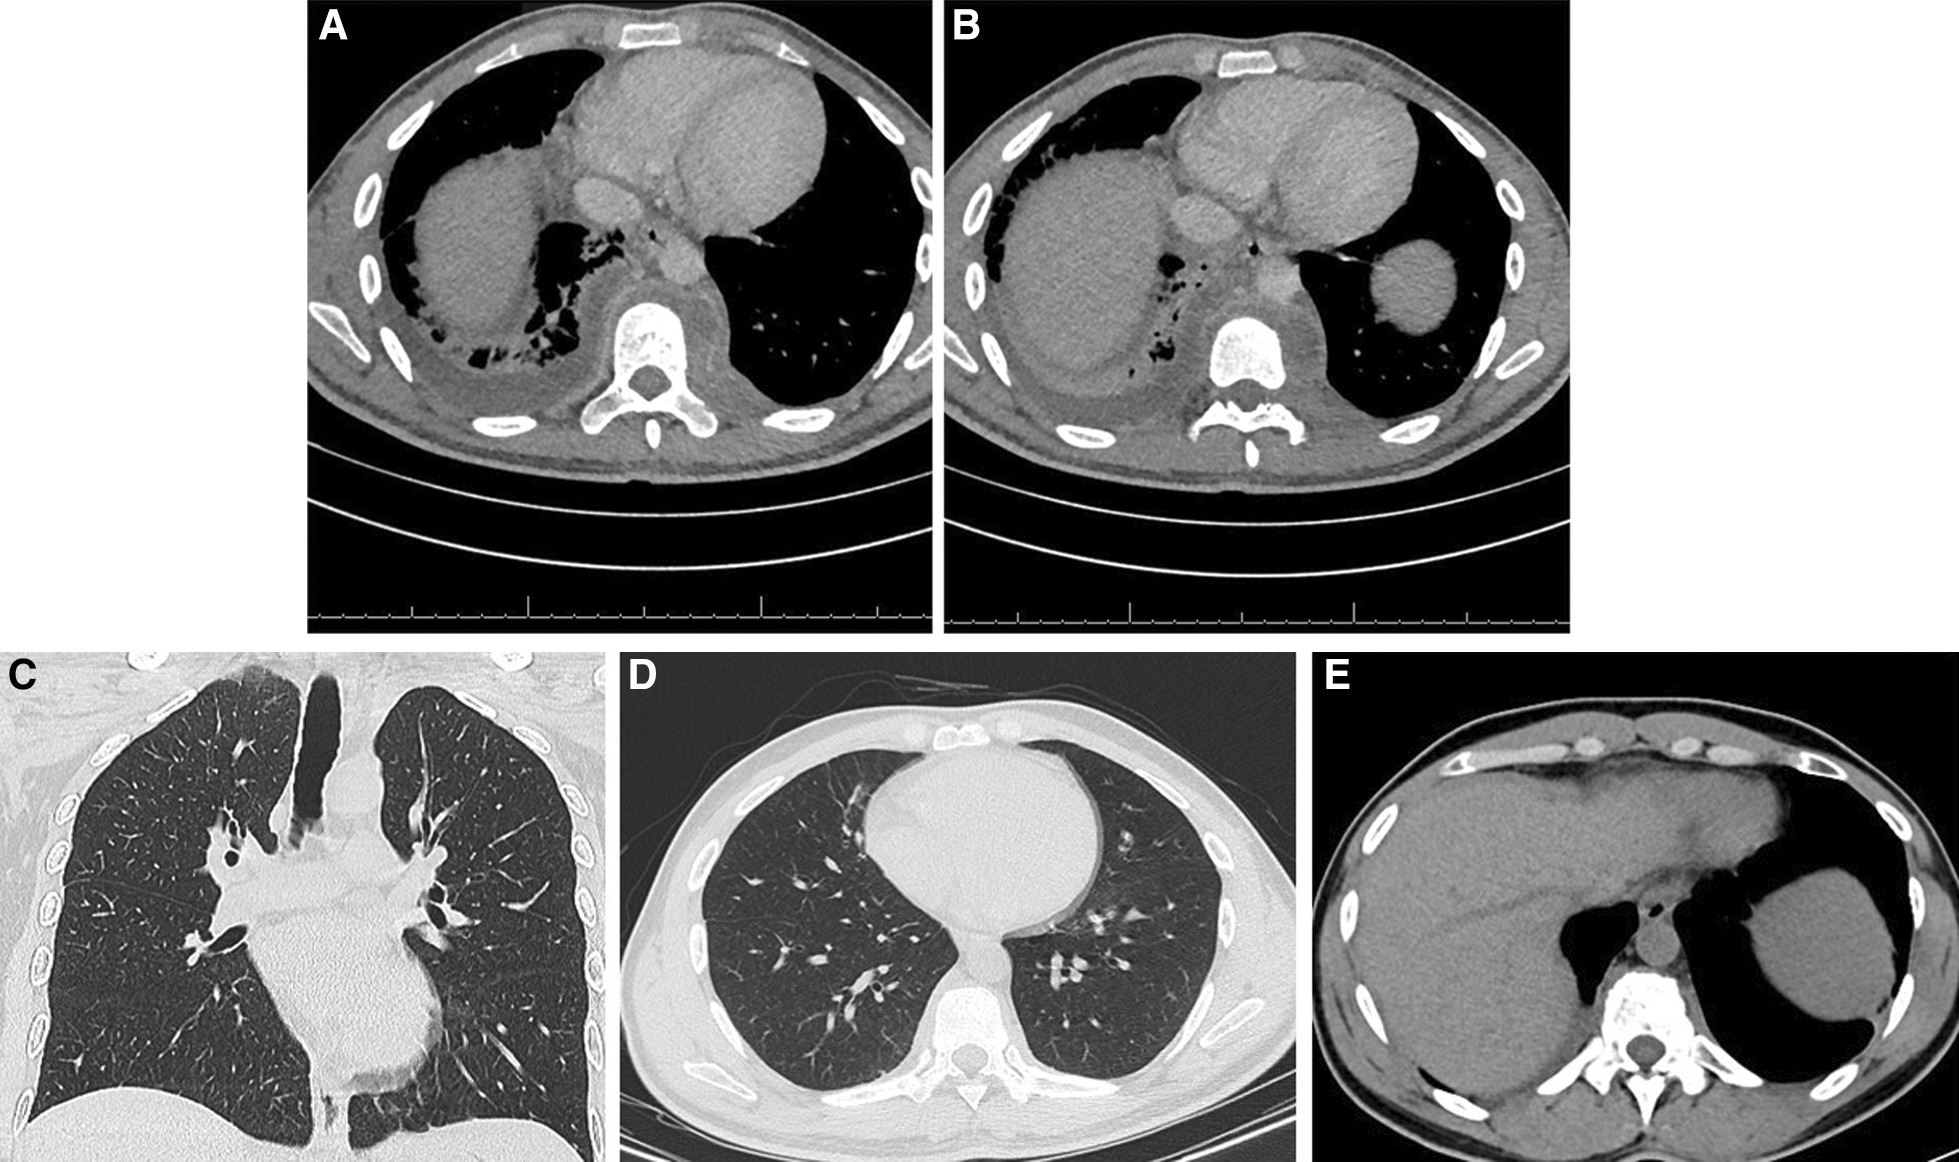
a, b Chest CT scan showing bilateral effusions more over the right, right segmental infiltrates, and loculated paraspinal collections. c, d, and e Follow up CT scans of the chest showing complete resolution of the empyema and paraspinal collections
